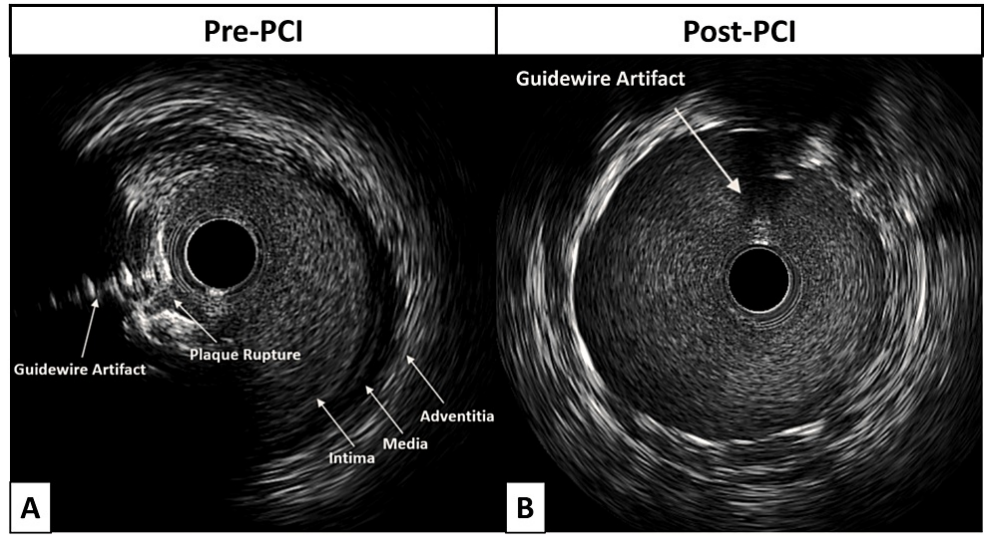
(A) Intravascular ultrasound (IVUS) of the proximal left anterior descending (LAD) artery shows a significant plaque burden, confirming plaque rupture as the cause of the patient's ST-elevation myocardial infarction (STEMI). (B) The IVUS post-percutaneous coronary intervention (PCI) shows adequate stent expansion and apposition, with no edge dissections.
